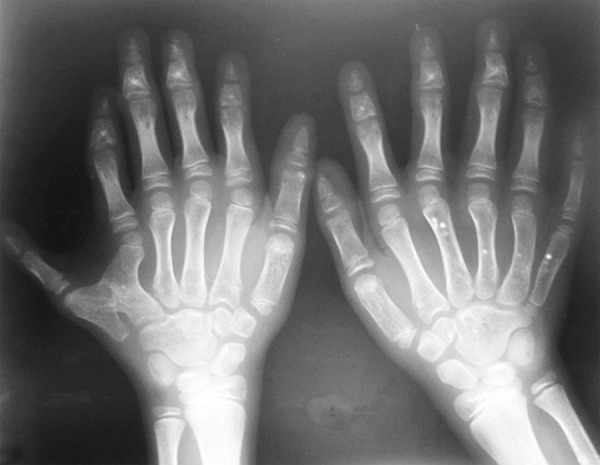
Radiograph showing postaxial polydactyly
